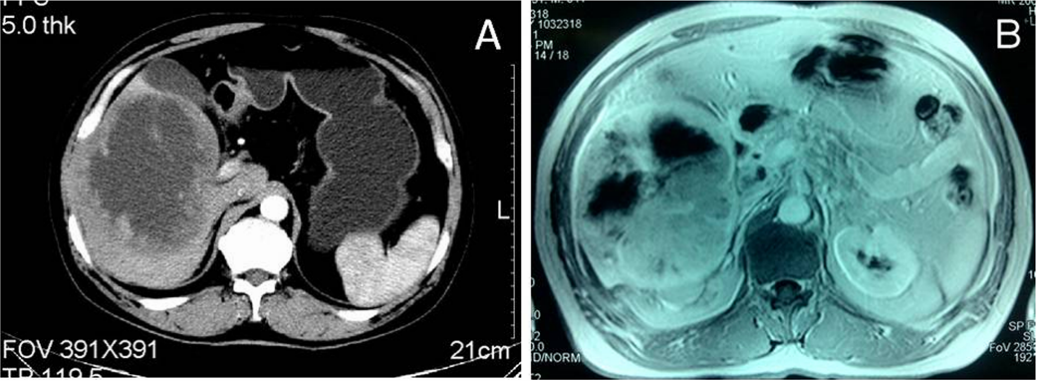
Imaging findings of case 2. (A), (B) Computed tomography and magnetic resonance imaging showing multiple lesions in the right liver lobe.
